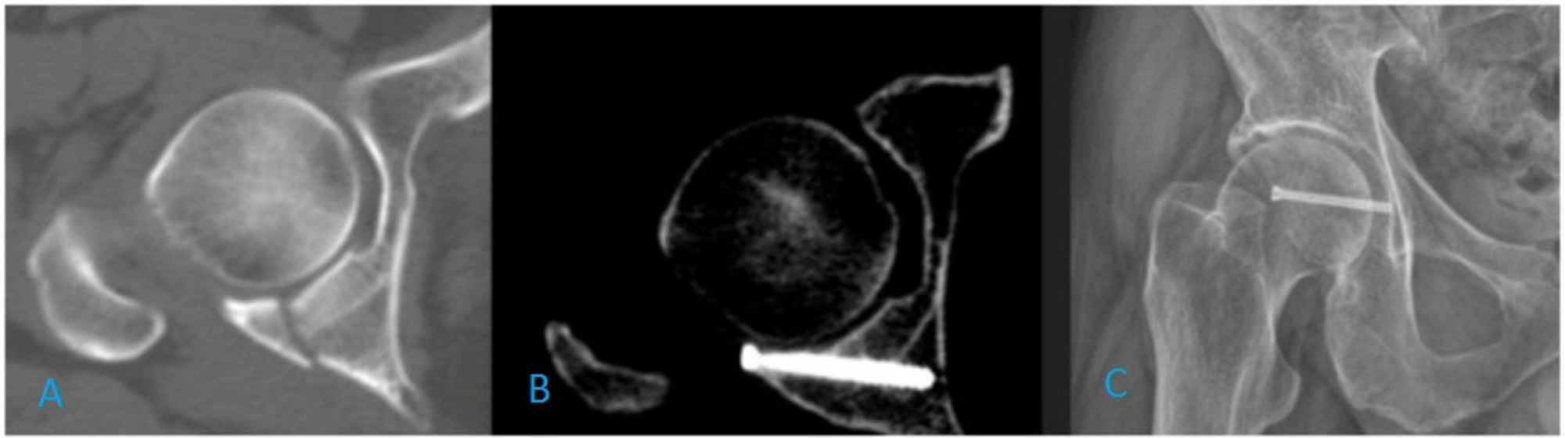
A: Preoperative axial CT scan image of the hip. B: Postoperative first year axial CT scan of the hip. C: Postoperative first day X-ray of the hip.
